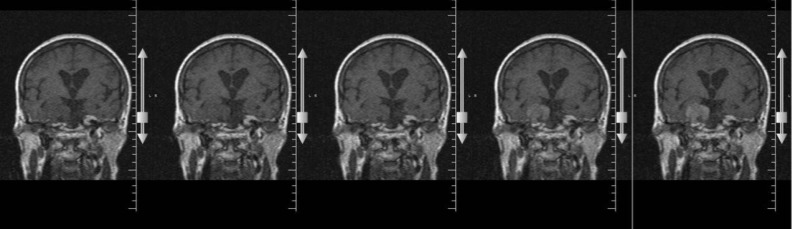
Contrast (Gadolinium) enhanced MRI (T2-weighted) of the patient which shows a CP angle tumor with enhancement compatible with meningioma
